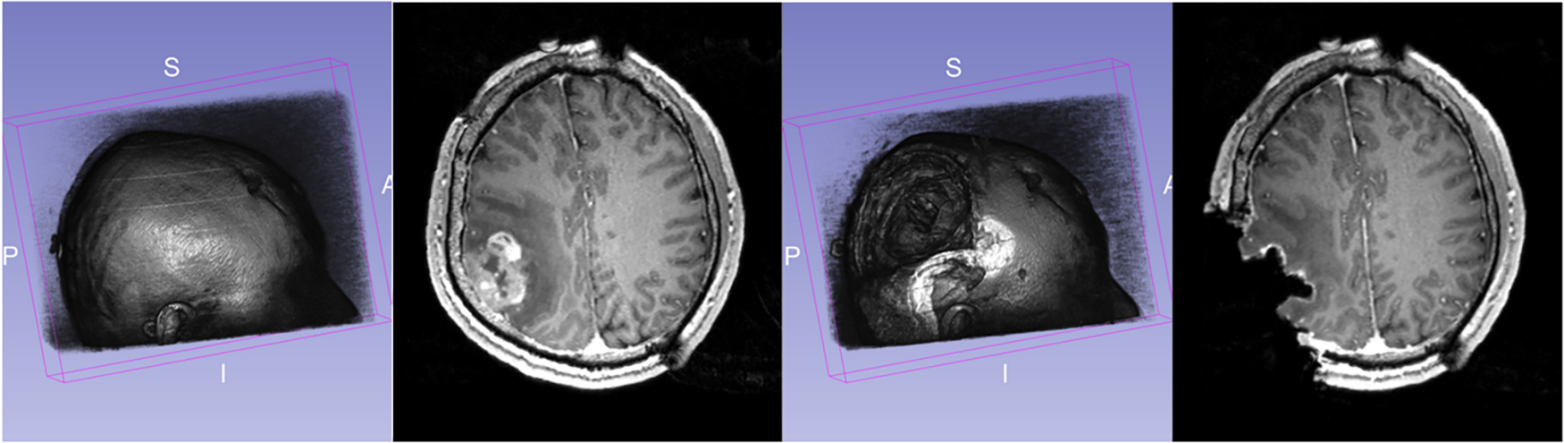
Discrepancies between preoperative and intraoperative MRI before and during neurosurgery: volume rendering and axial view. Preoperative MRI (left) and intraoperative MRI (right) are acquired after a substantial part of the tumor is removed.
